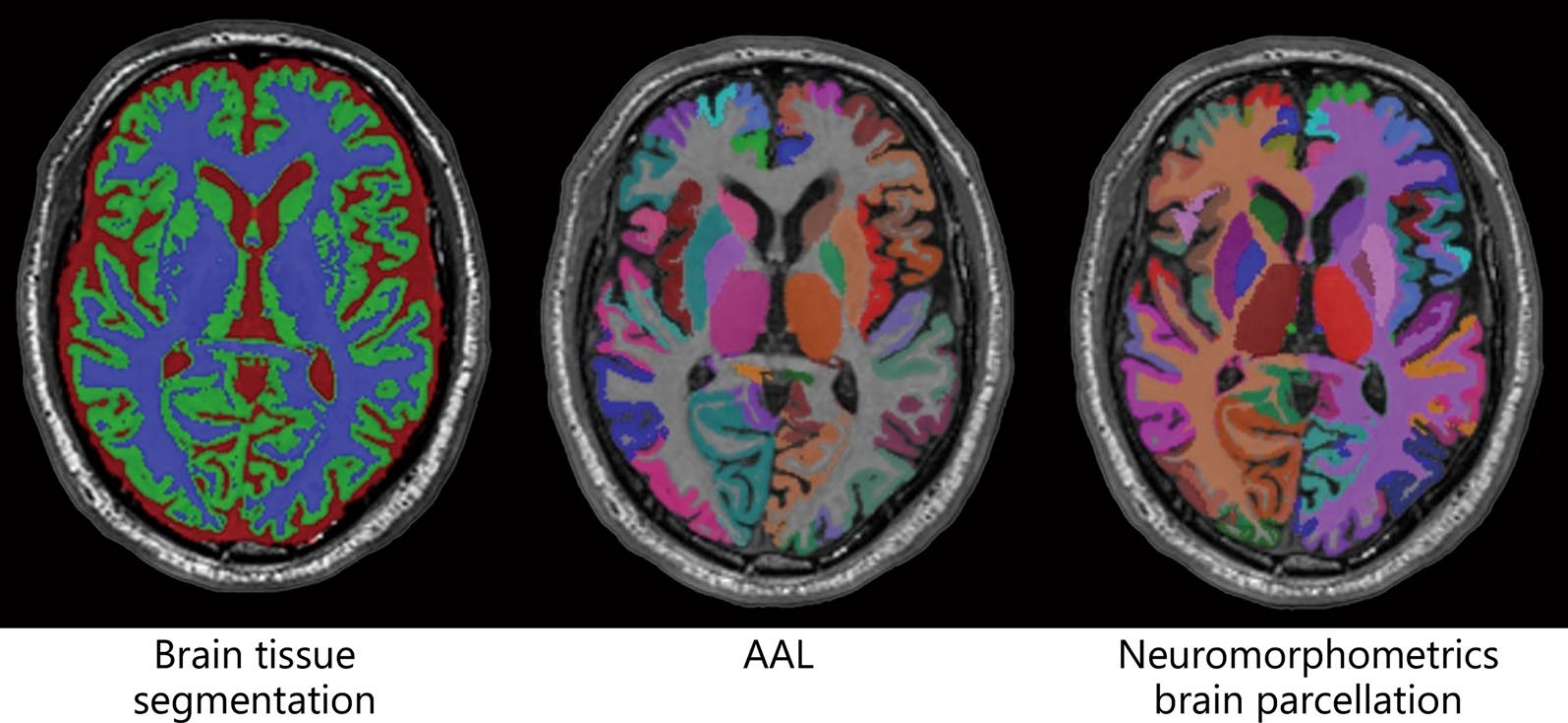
Axial cross-sections showing example brain segmentation and parcellation labels overlaid on the structural MRI. Brain tissue segmentation (left), coloured brain regions defined in the automated anatomical labeling (AAL) atlas (middle), coloured brain regions defined in the Neuromorphometrics atlas (right). AAL Automated anatomic labeling
